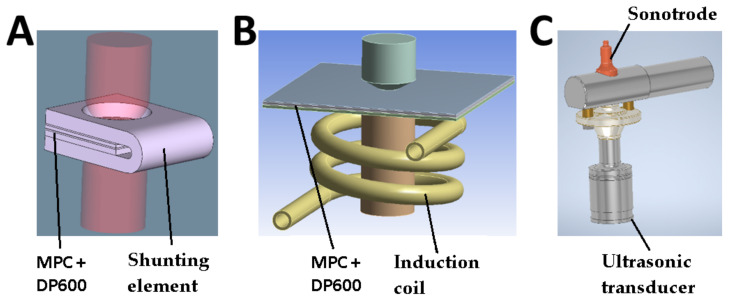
The overview of the model geometries: (A) shunt current-assisted RSW; (B) induction heating-assisted RSW; and (C) ultrasonic-assisted RSW.
